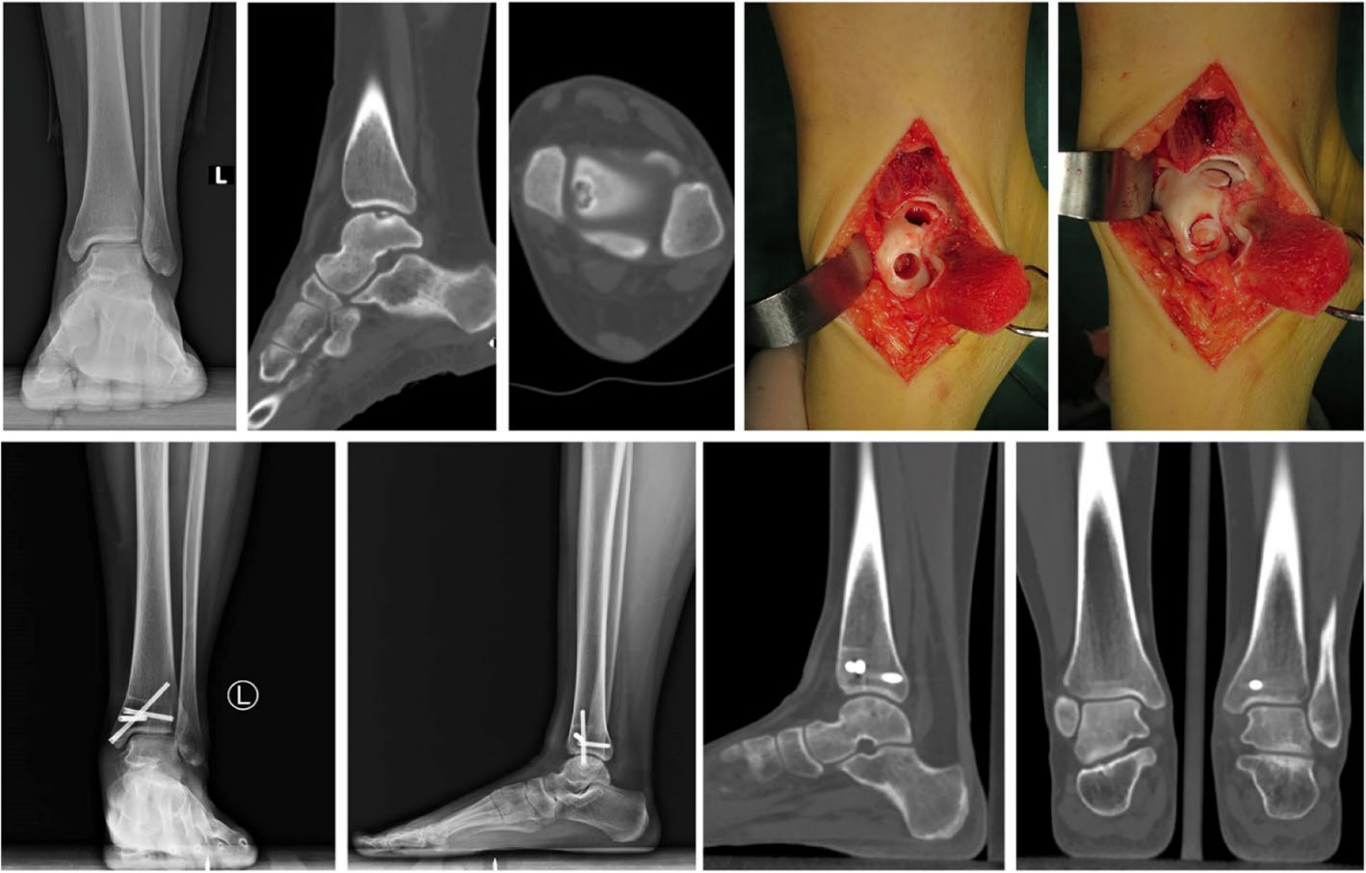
Case2, female, 41y, osteochondral lesions of left talus. A-C: preoperative imaging (X - ray and CT) supported the diagnosis; D-E: the lesion was exposed through the medial malleolus triplane osteotomy and AOT for OLTs from a non-weight-bearing area, and the donor defect was filled with a bone graft with periosteum from the tibia; F-I: at 1.5-year follow-up, imaging showed that the grafts had fused with the original bone in the talus, and the medial malleolus had achieved anatomic union
